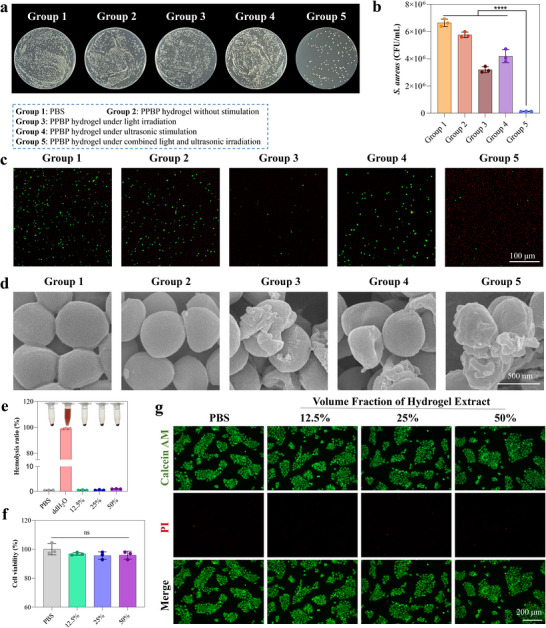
In vitro antibacterial efficacy and biocompatibility evaluation of PPBP hydrogel. (a) Representative photographs of *S. aureus* colonies on agar plates after treatment with PBS (Group 1), PPBP hydrogel (Group 2), PPBP and light irradiation (Group 3), PPBP and ultrasonic stimulation (Group 4), and PPBP with combined light and ultrasonic stimulations (Group 5). (b) Quantitative analysis of *S. aureus* viability (CFU/mL) corresponding to treatments in (a). Data are presented as mean ± S.D. (*n* = 3, ^^
